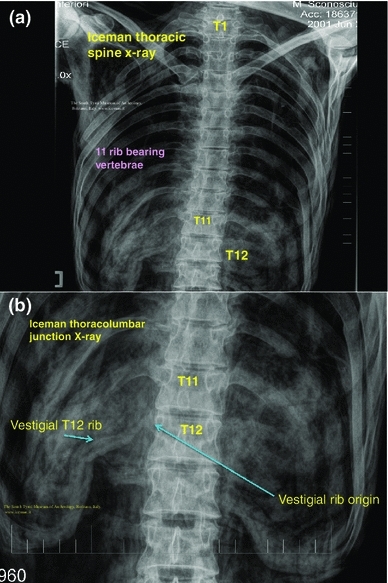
a Iceman thoracic spine X-ray which shows the thoracic vertebrae with 11 normal sized *right* and *left* ribs. The location of thoracic (T) vertebrae 1, 11 and 12 are shown as T1, T11, T12, b the X-ray of the lower thoracic spine and upper lumbar spine known as the thoracolumbar junction is shown to identify origin and position of the *right* thoracic 12 vestigial rib
